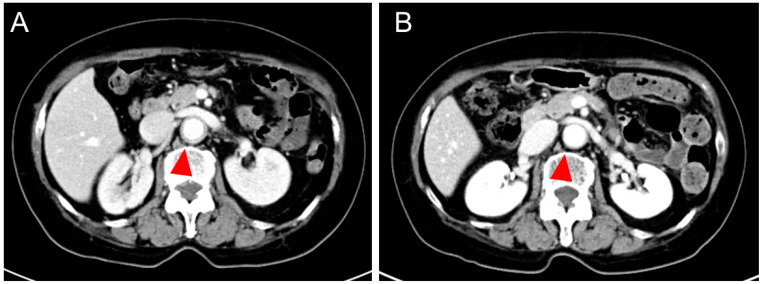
Contrast-enhanced CT at onset of disease (day 24 after vaccination) in 2021 (A) showed thickening of the aortic vessel wall (arrows). Contrast-enhanced CT on day 22 of treatment (day 63 after vaccination) (B) showed improvement in thickening of the aortic vessel wall (arrows).
